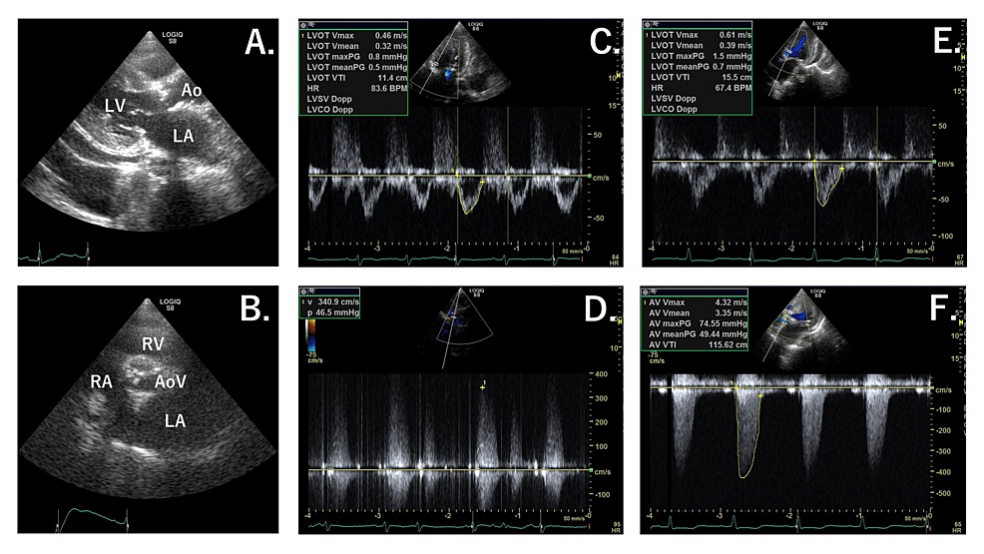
Echocardiography of the patient (A and B: day 1, C and D: day 11, E and F: day 31) Day 1 (A and B): Parameters related to Doppler (LVOT-VTI, AV-VTI) could not be measured because of difficulties by highly calcified aortic valve and patient positioning. Day 11 (C and D): Echocardiography measurements demonstrate a peak transvalvular gradient of 46.5 mmHg and aortic valve velocity of 3.41 m/sec. AVA was 0.37 cm^2^ by planimetry. AV-VTI could not be measured. Day 31 (E and F): Echocardiography measurements demonstrate mean gradient of 49.44 mmHg, maximum gradient of 75 mmHg, mean aortic valve velocity of 3.4 m/sec, and maximum aortic valve velocity of 4.3 m/sec. AVA is 0.39 cm^2^ by planimetry and 0.89 cm^2^ by continuous equation. Her vital parameters at day 31 were as follows: blood pressure, 136/67 mmHg; heart rate, 65 bpm, sinus rhythm. She had no severe anemia (hemoglobin: 10.0 g/dL and hematocrit: 31.8%). LA: left atrium, LV: left ventricle, RA: right atrium, RV: right ventricle, Ao: aorta, AoV: aortic valve, LVOT: left ventricular outflow tract, VTI: velocity time integral, AV: aortic valve, AVA: aortic valve area
